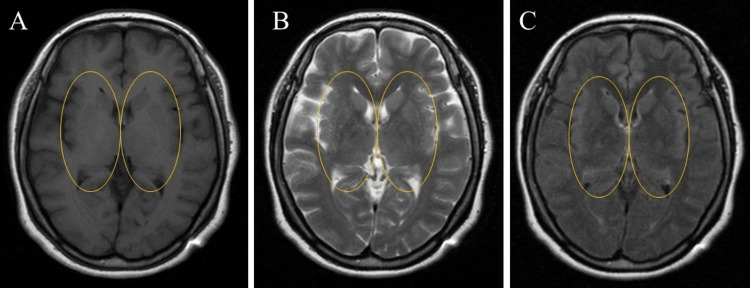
Brain MRI at the level of the basal ganglia Brain MRI findings were normal. There are no abnormalities in the basal ganglia (striatum, globus pallidus, subthalamic nucleus, substantia nigra), thalamus, or posterior limb of the internal capsule (circled areas). A: T1 weighted image; B: T2 weighted image; C: fluid attenuated inversion recovery (FLAIR) image
